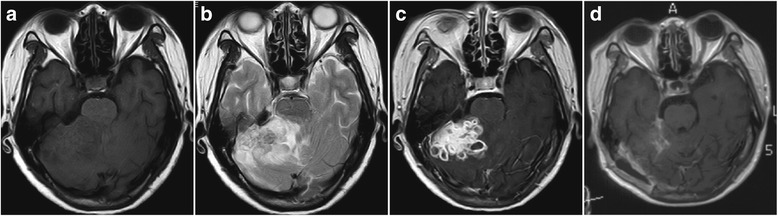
Typical MRI of intracisternal tuberculoma. a The lesions showed iso- and hypo-intense on T1WI. b Mixed signal intensity on T2WI. c Contrast-enhanced T1WI sequence showed multiple ring enhancing lesions. d The postoperative MRI of the patient
